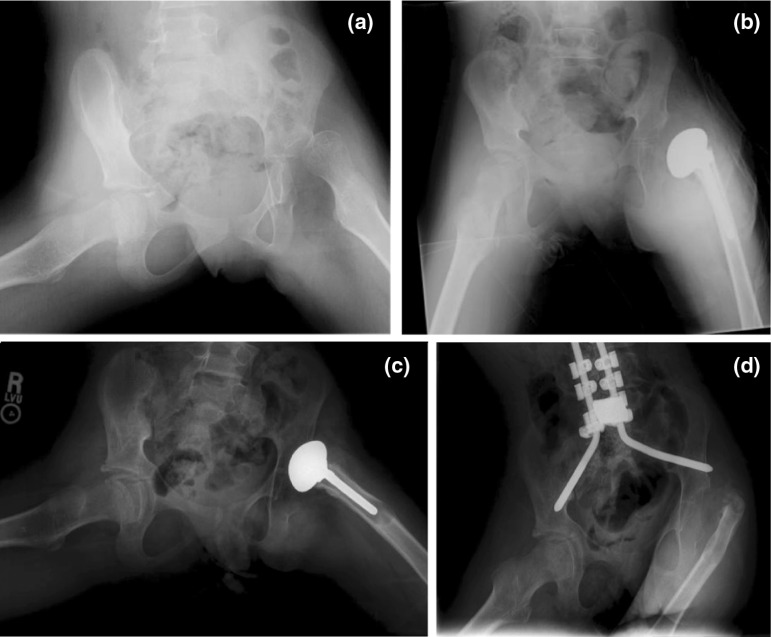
a Pre-operative radiograph of a 9-year-old child who required revision surgery 15 months after proximal femur prosthetic interpositional arthroplasty (PFIA) secondary to severe pain and osteolysis. b Immediate post-operative radiograph after PFIA. c 11-month post-operative radiograph showing osteolysis surrounding the proximal femoral prosthesis. d Radiograph showing complete prosthesis removal 15 months after PFIA. Concomitant posterior spinal fusion with instrumentation was completed at time of prosthesis removal
